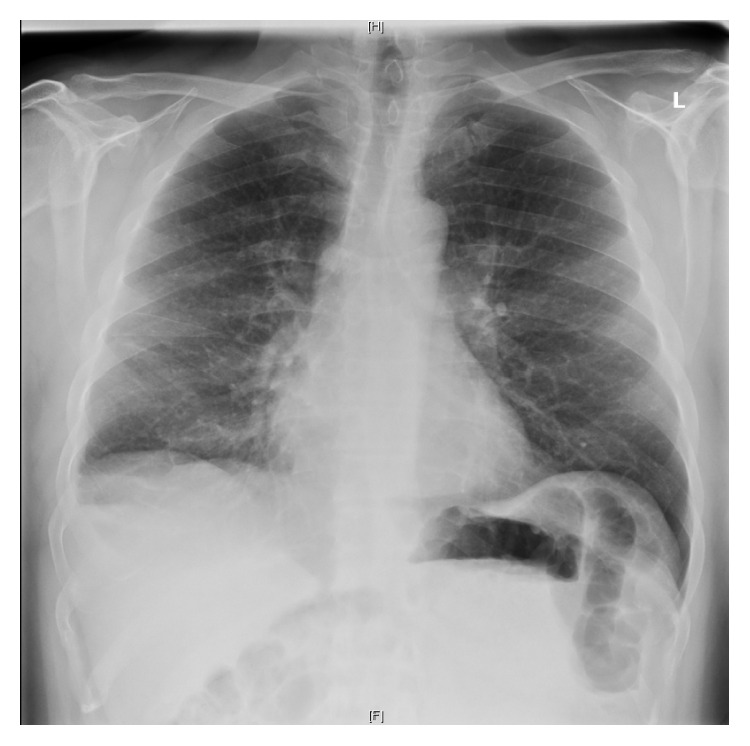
Chest radiography 6 months after diagnosis showing drastic improvement. The lungs were clear except for a small persistent right-sided pleural effusion. The cardiothoracic ratio and the mediastinal contours were unremarkable.
